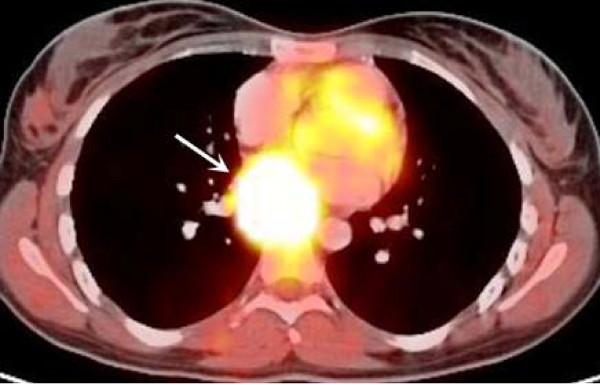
Axial view of PET-CT scan image demonstrates a large hypermetabolic mass with intense uptake of F^18 ^Levo-DOPA in the posterior mediastinum near the left atrium which is consistent with paraganglioma.
